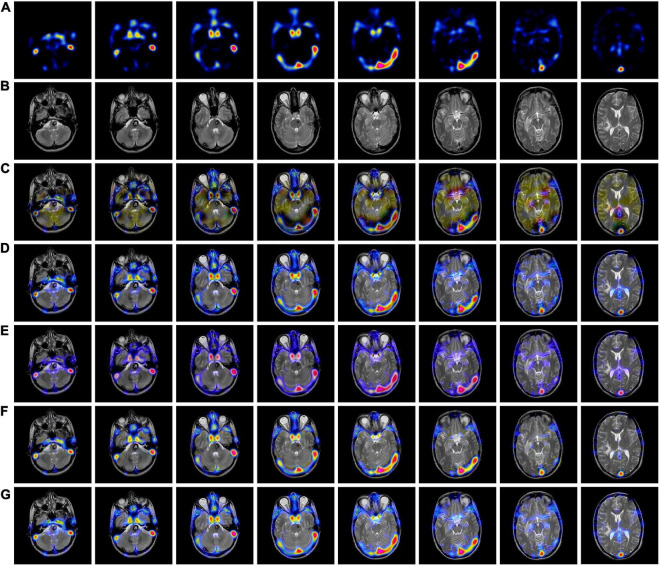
MRI-SPECT fusion images of the five methods in Paras1. (A) Single photon emission computed tomography (SPECT) source image; (B) magnetic resonance imaging (MRI) source image; (C) nonsubsampled contourlet (NSCT) fusion result; (D) non-subsampled shear wave transform (NSST) fusion result; (E) guided filter ng fusion (GFF) fusion result; (F) Laplacian redecomposition (ReLP) fusion result; (G) fusion result of the proposed method.
